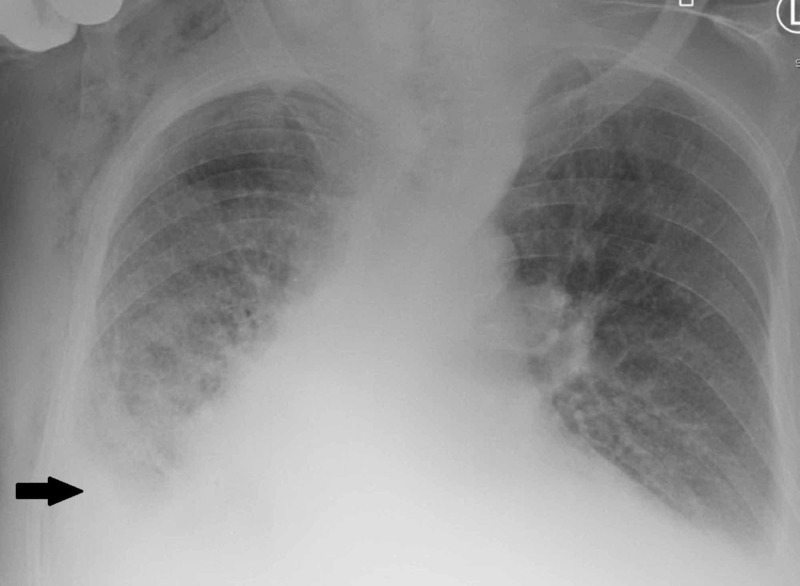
Posteroanterior radiograph showing decrease of right sided pleural effusion volume after pleurodesis (arrow)
